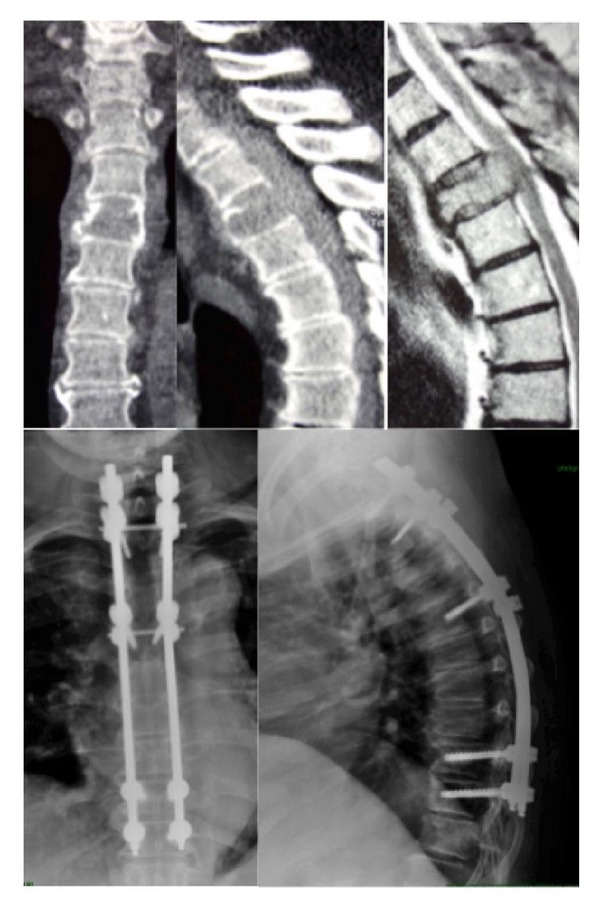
CT scan and MRI of a 71-year-old, obese female (120 Kg) with osteolysis of the T4. Hybrid construct performed percutaneously in the lower tract (T9-10) and by open approach in upper part (T1-2/5). No failure of the implant at 1-year follow-up X-rays.
